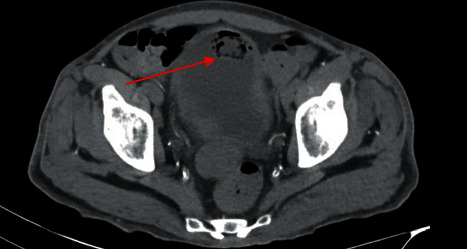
Transverse abdominal CT image showing evidence of bladder fungal ball with emphysematous cystitis (red arrow).
